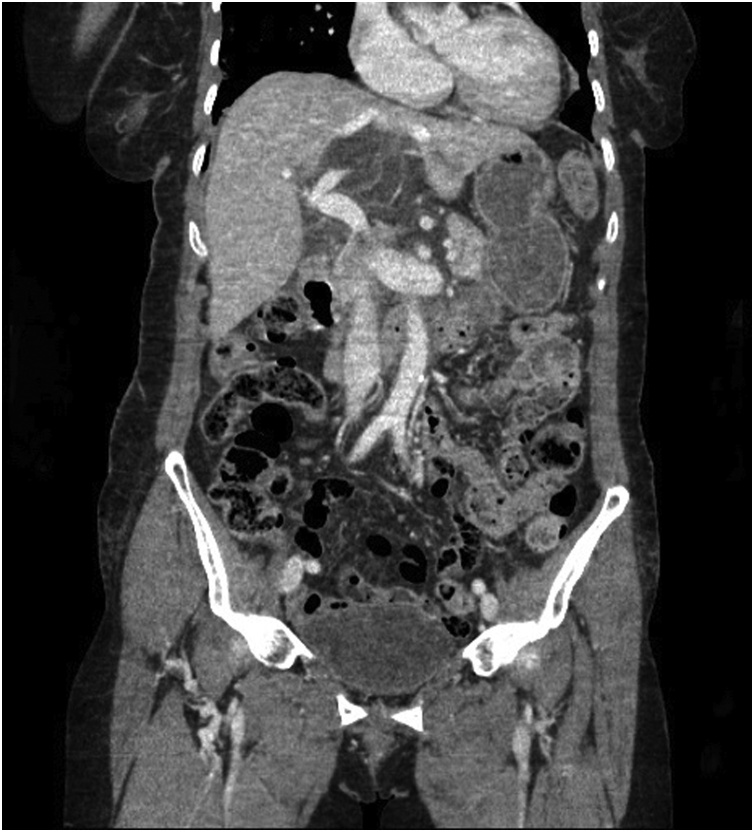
Coronal view on an abdominal computed tomography scan of the patient, showing fluid collection and a cystic lesion around the caudate lobe of the liver.
